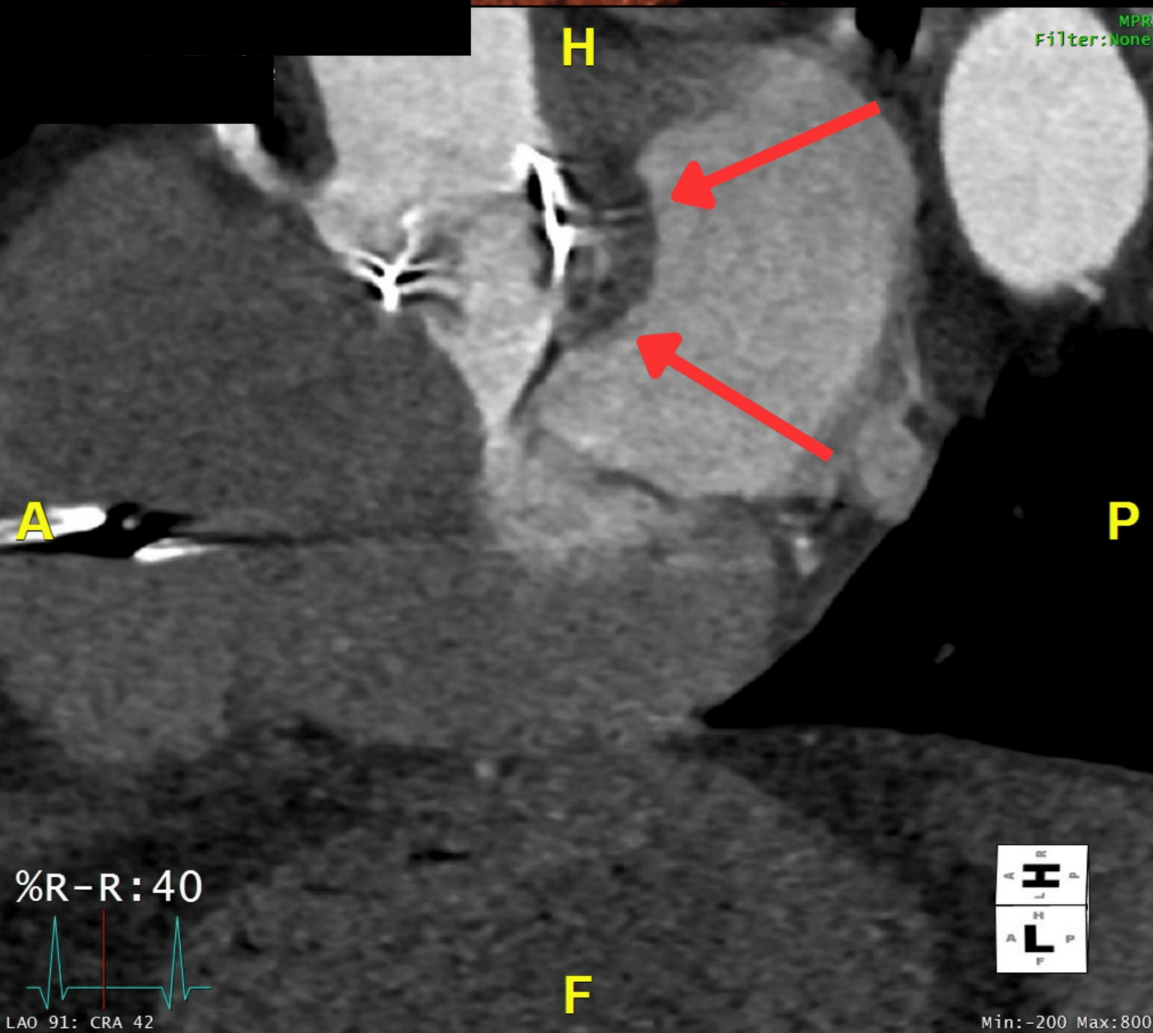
CT cardiac coronary angiogram showing aortic root abscess (red arrows).
